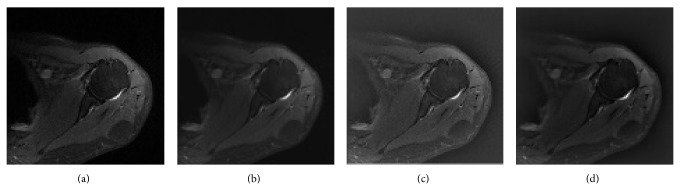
(a) Orginal PD weighted MRI, (b) image obtained after SRAD method, (c) result of the homomorphic filter, and (d) result obtained after using SRAD method and homomorphic filter.
